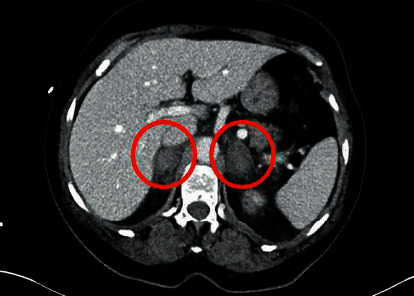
CT of the abdomen at admission (horizontal plane).
